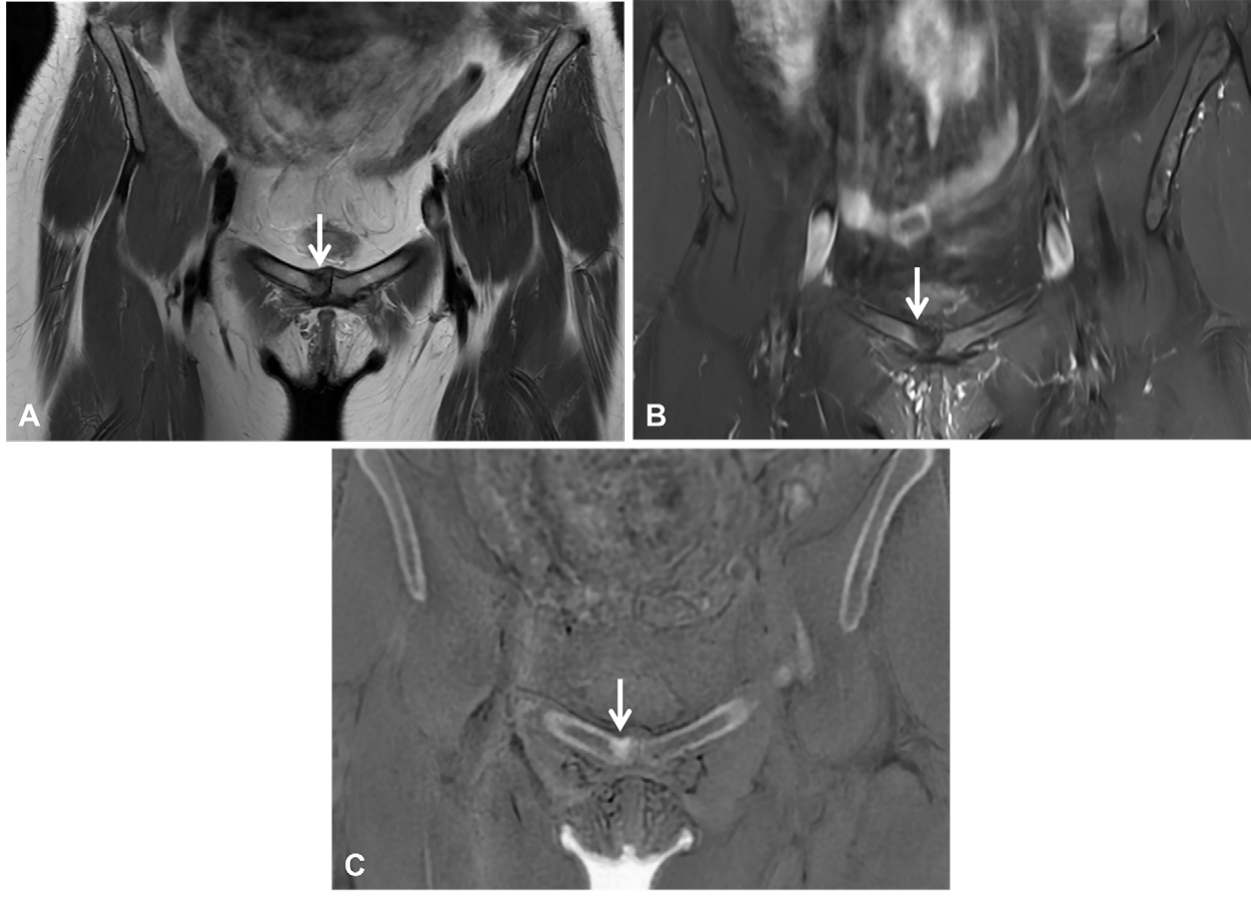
MRI of the pelvis in a 48-year-old female long-distance runner demonstrating a stress injury (arrows). (A, B) Coronal T1 and proton-density fat-suppressed images demonstrate signal changes in the pubic symphysis with associated bone marrow edema (arrow). (C) The coronal ZTE image demonstrates a sclerotic line compatible with a stress injury.
